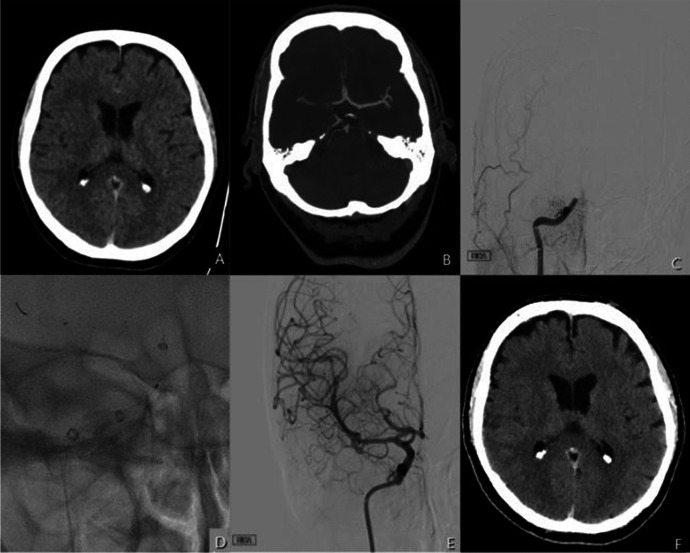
A patient in their 60 s presented with an NIHSS score of 12 and ASPECT score 6 A. After CTA confirmed an occlusion of the right terminal ICA and right proximal M1 B, the patient was transferred for MT. Initial angiography confirmed an abrupt filling defect of the right terminal ICA (C). A NeVa Net 4.0 × 30 mm was deployed for 5 min per our standard practice D. Angiography demonstrated eTICI 3 recanalization after the first pull E. The 24 h CT scan demonstrates expected hypodensity within the basal ganglia (ASPECT score 6) with no extension of the infarction when compared to the pre-procedural CT F
